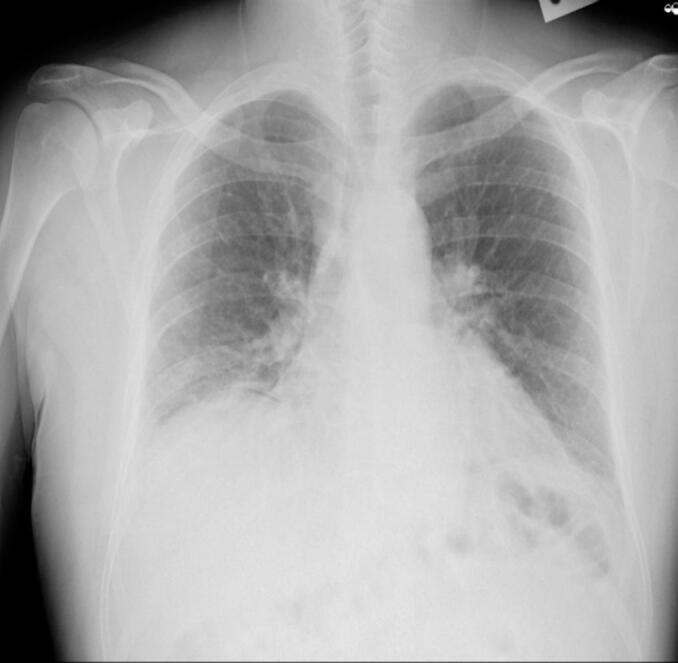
Chest X-ray showing no significant findings.
